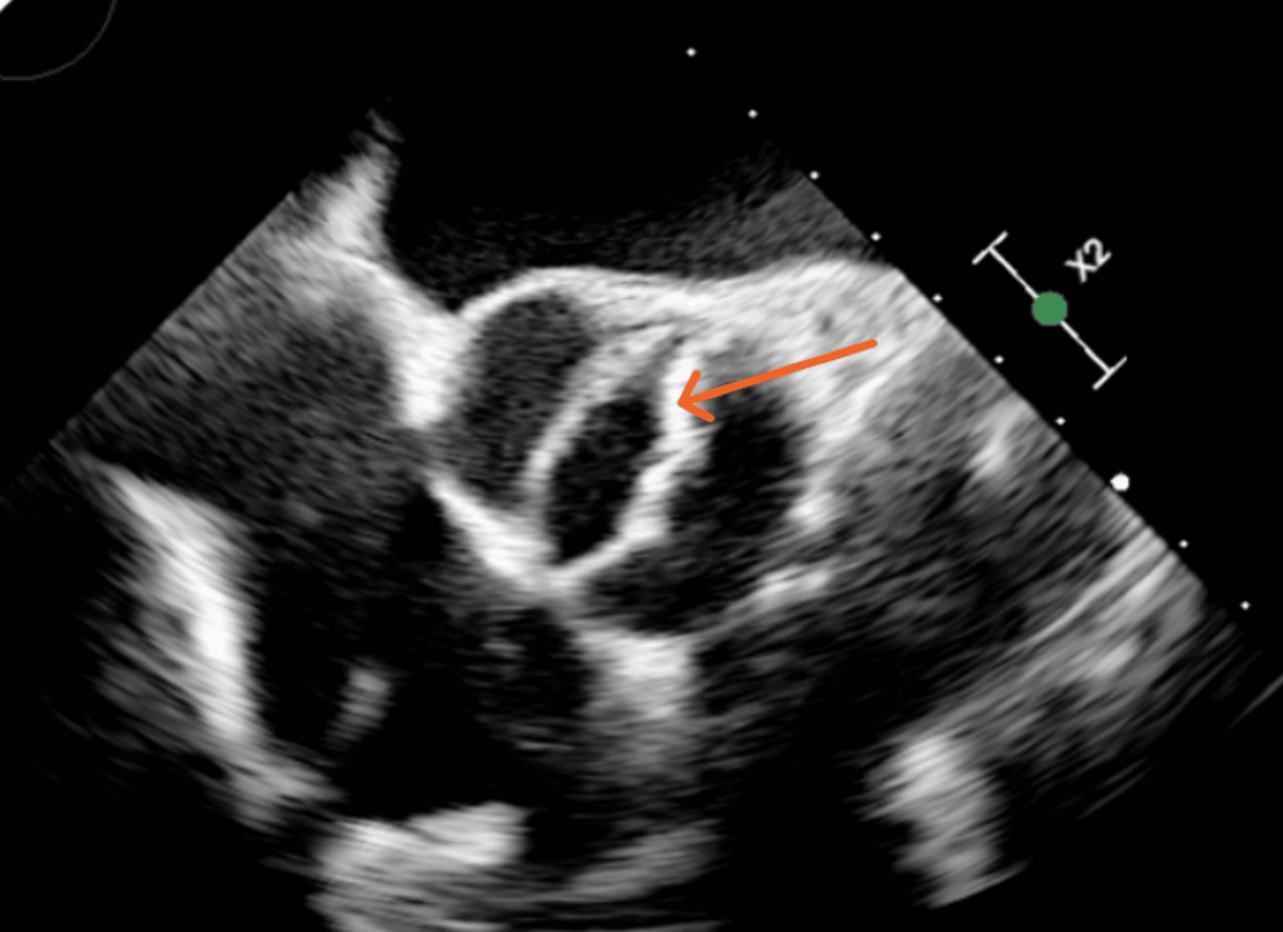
Parasternal short-axis TTE view demonstrating a BAV with fusion of the right and left coronary cusps (red arrow). The fused commissure produces the characteristic elliptical systolic opening pattern associated with bicuspid valve morphology X2 denotes a two-fold zoom applied by the echocardiography machine, indicating image magnification rather than an anatomical distance measurement. BAV: bicuspid aortic valve; TTE: transthoracic echocardiogram
